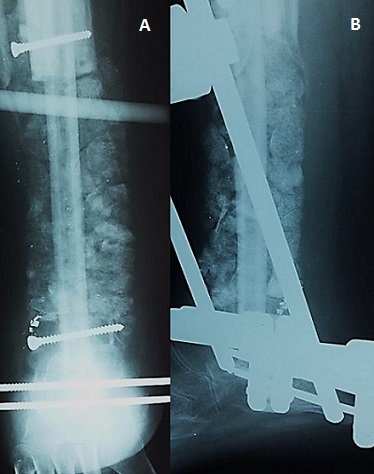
(A, B) plain radiographs post reconstruction
